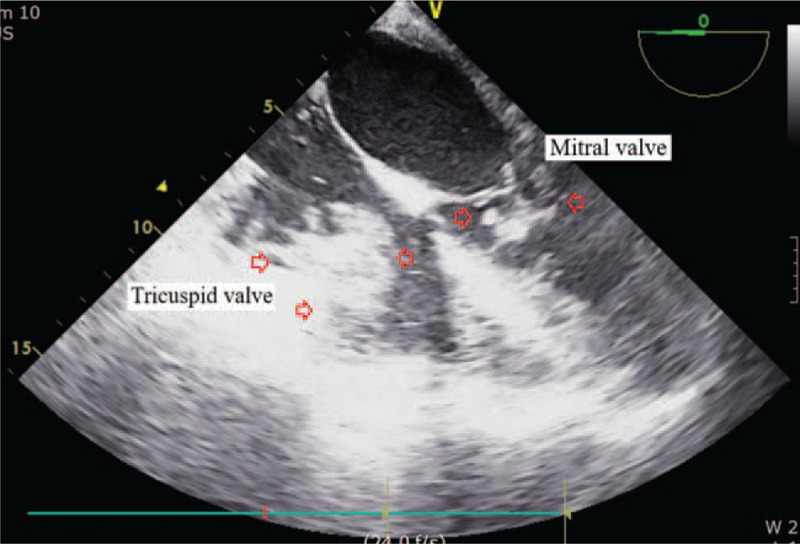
Multiple, huge thromboembolic clots on the tricuspid and mitral valves, seen on transesophageal echocardiography. Arrows point to the thromboembolic clots.
